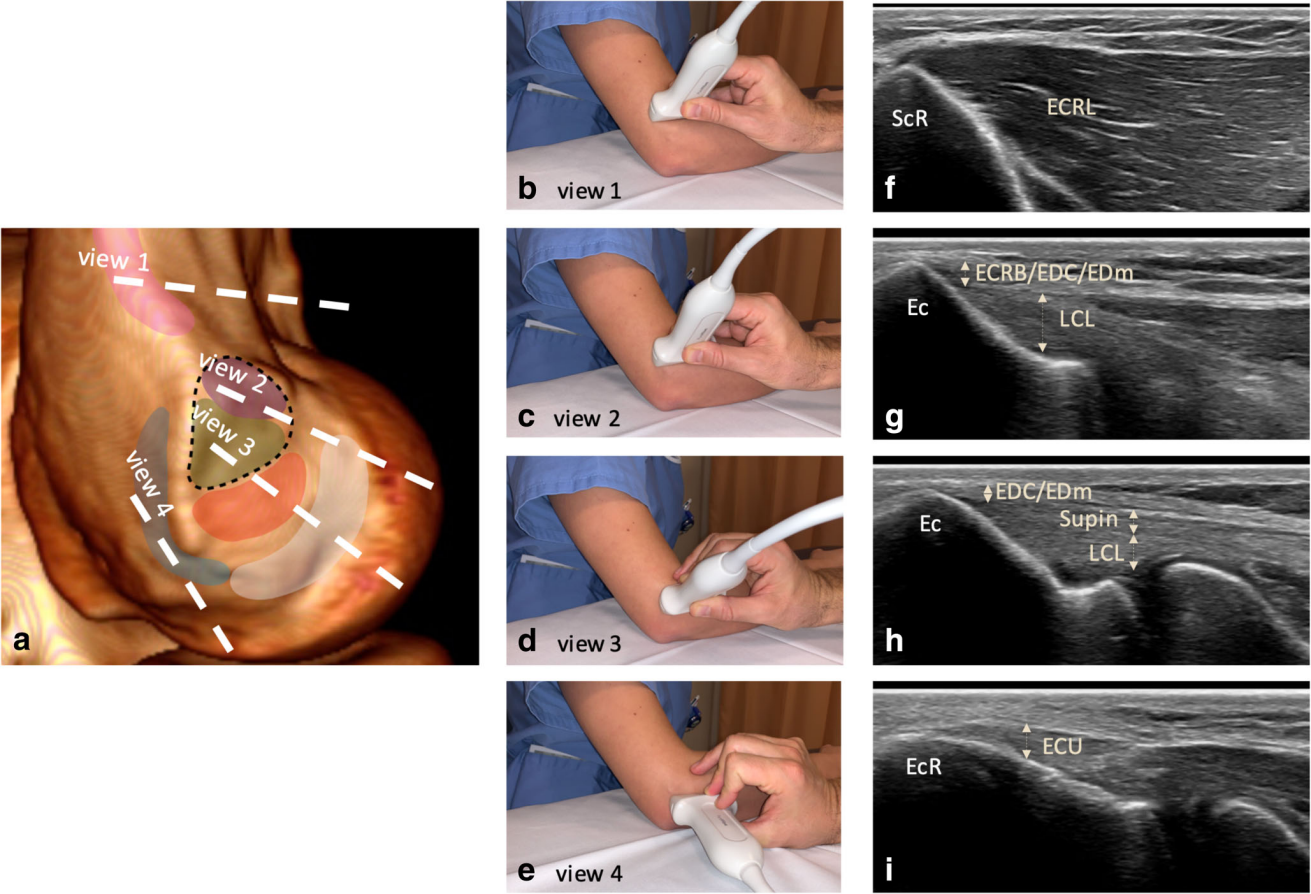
Ultrasonographic assessment technique for the lateral epicondyle with position of the different ultrasonographic views on the lateral epicondyle shown on a CT with surface rendering (a), on photographs of a volunteer (b–e), with corresponding ultrasonographic images (f–i): View 1: supracondylar ridge (easily identified in the short-axis plane of the distal humerus): the muscular attachment of the ECRL onto the supracondylar ridge is identified. View 2: anterosuperior aspect of epicondyle (slightly moving the probe distally to the epicondyle from View 1, at its anterior aspect): the conjoint ECRB/EDC/EDm tendon is visible. View 3: intermediate view (between views 2 and 4): from superficial to deep, the EDC/EDm tendons, supinator tendon and LCL are visible. View 4: posterior aspect of epicondylar ridge: From surface to deep, the ECU tendon can be easily identified. ECRL: Extensor carpi radialis longus, ECRB: extensor carpi radialis brevis, EDC: extensor digitorum communis, EDm: extensor digiti minimi, Supin: supinator tendon, ECU: extensor carpi ulnaris, LCL: lateral collateral ligamentous complex, ScR : supracondylar ridge, Ec : epicondyle, EcR : epicondylar ridge
